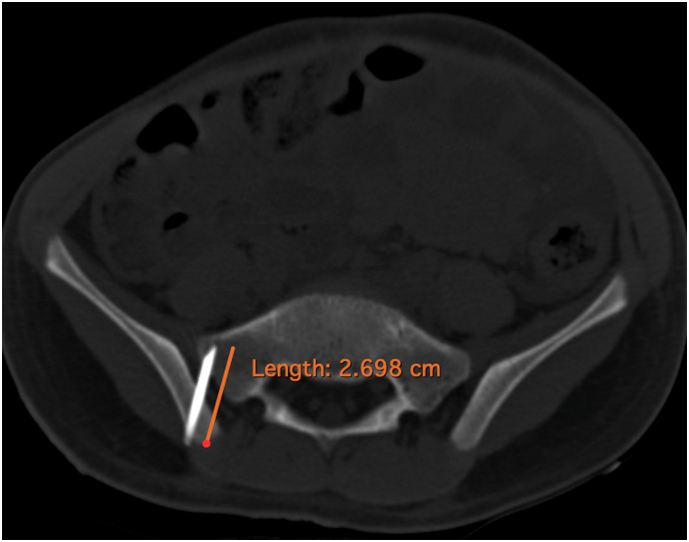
CT scan image of the foreign body measuring about 2.7 cm in length penetrating the right sacroiliac joint.
